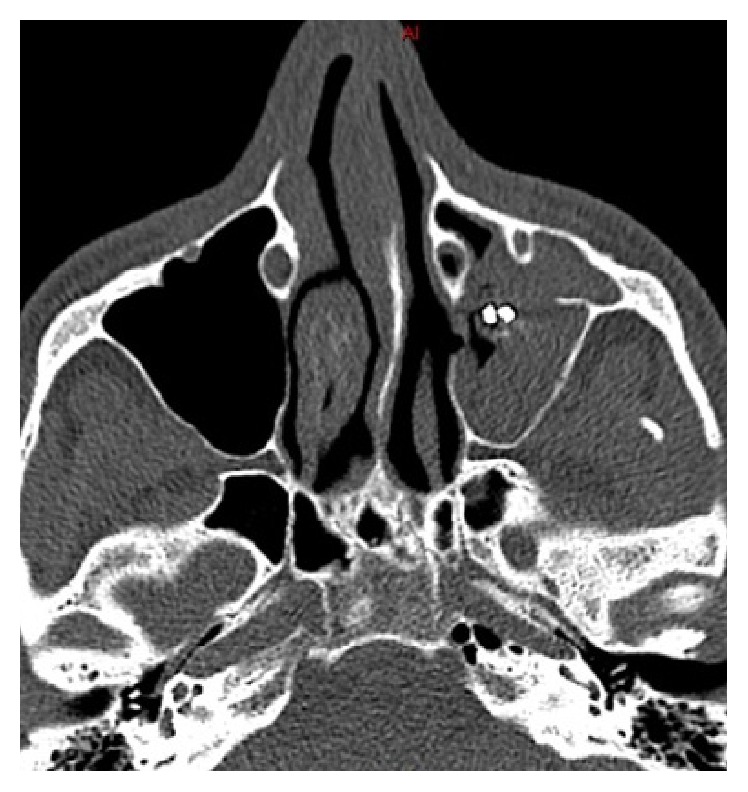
CT facial scan that shows the aspect of an endosinusal foreign body.
